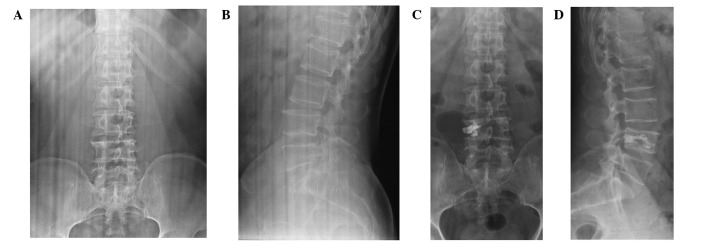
Plain radiographs of the lumbar spine, (A and B) preoperative and (C and D) 6 months postoperative.
